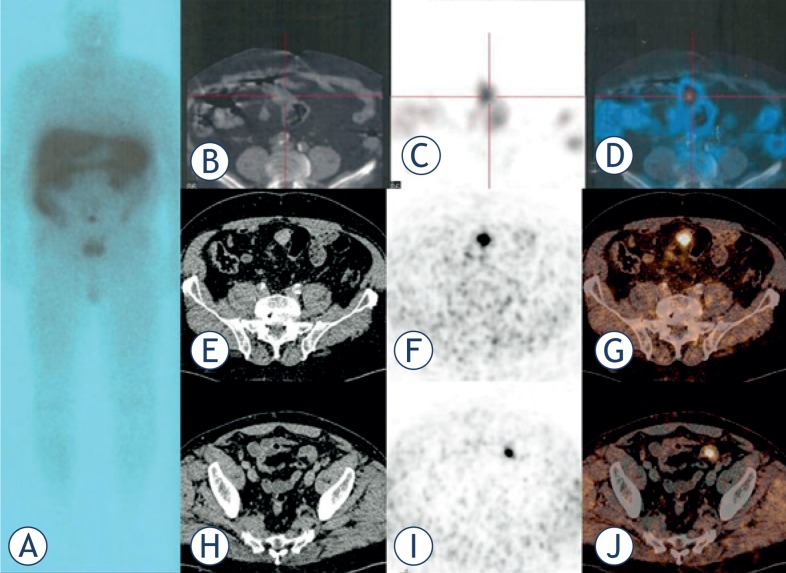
(A) In-111 DPTA octreotide detected one suspicious lesion located in the ileum (B–D) in a patient with NET liver metastases. Ga-68 DOTATOC PET/CT confirmed the lesion (E–G), but could visualise also an additional lesion in the ileum undetected by In-111 DTPA octreotide (H–J).
